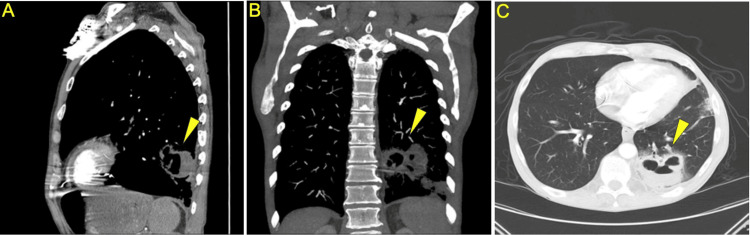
Cavitary lesion in left lower lobe. (A) Sagittal CT angiogram chest with contrast. (B) Coronal CT angiogram chest with contrast. (C) Apex to base CT angiogram chest with contrast.
